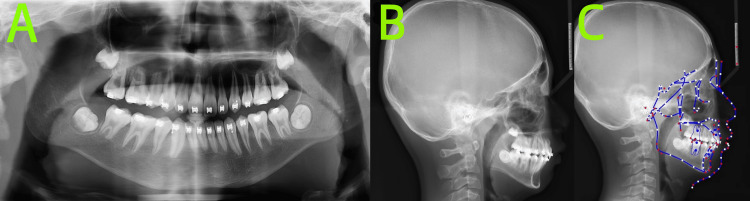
Post-treatment panoramic radiographs after Phase II A: Panoramic radiograph revealed no significant root resorption and good root parallelism throughout the dentition; B: Lateral cephalogram; C: Cephalometric tracing indicated significant retraction of both the upper and lower anterior teeth. Cephalometric tracing in Figure 2C created using the UCeph software (version 3.1, UCeph Software, Chengdu, China).
